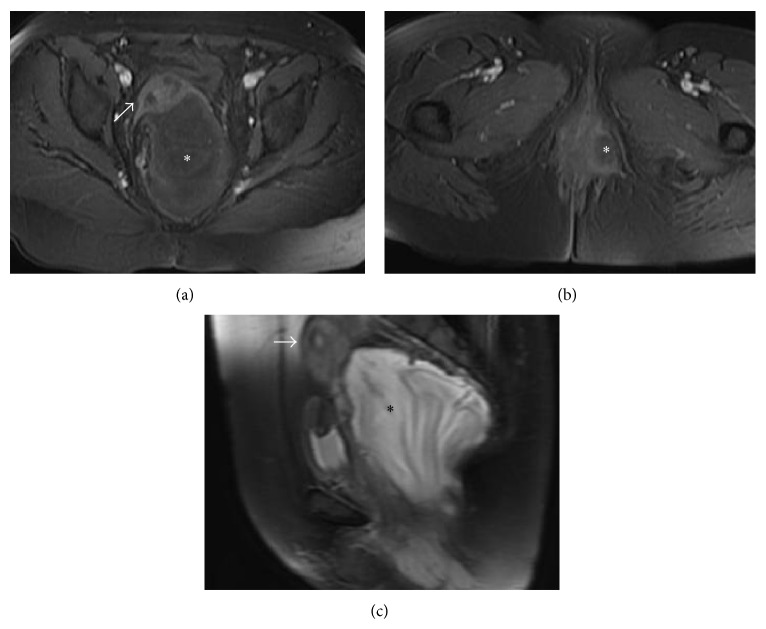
MR images of the lesion. (a, b) Axial, T1-weighted images with gadolinium enhancement and fat saturation. (c) Sagittal, T2-weighted image. The lesion (asterisk) has low signal on T1W images and high signal on T2W image. There is minimal contrast enhancement. The lesion causes anterior displacement of the uterus (arrows (a, c)). On (b), the downward extension of the lesion into the ischiorectal fossa is evident.
